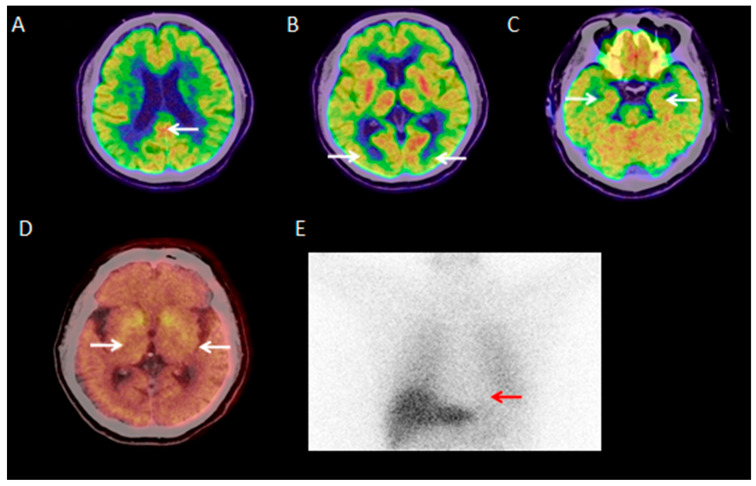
A 69-year-old male patient with a 5-year history of bradykinesia and unsteady gait, and visual hallucinations (reporting “fighting little devils” at night and perceiving hats/plastic bags as skulls). ^18^F-FDG PET/CT shows preserved posterior cingulate metabolism (posterior cingulate island sign), bilateral occipital hypometabolism, and preserved bilateral medial temporal metabolism ((A–C); white arrows). ^18^F-FP-CIT PET/CT shows reduced uptake in the bilateral putamen tails ((D); white arrow). ^131^I-MIBG scintigraphy indicates cardiac sympathetic denervation ((E); red arrow).
